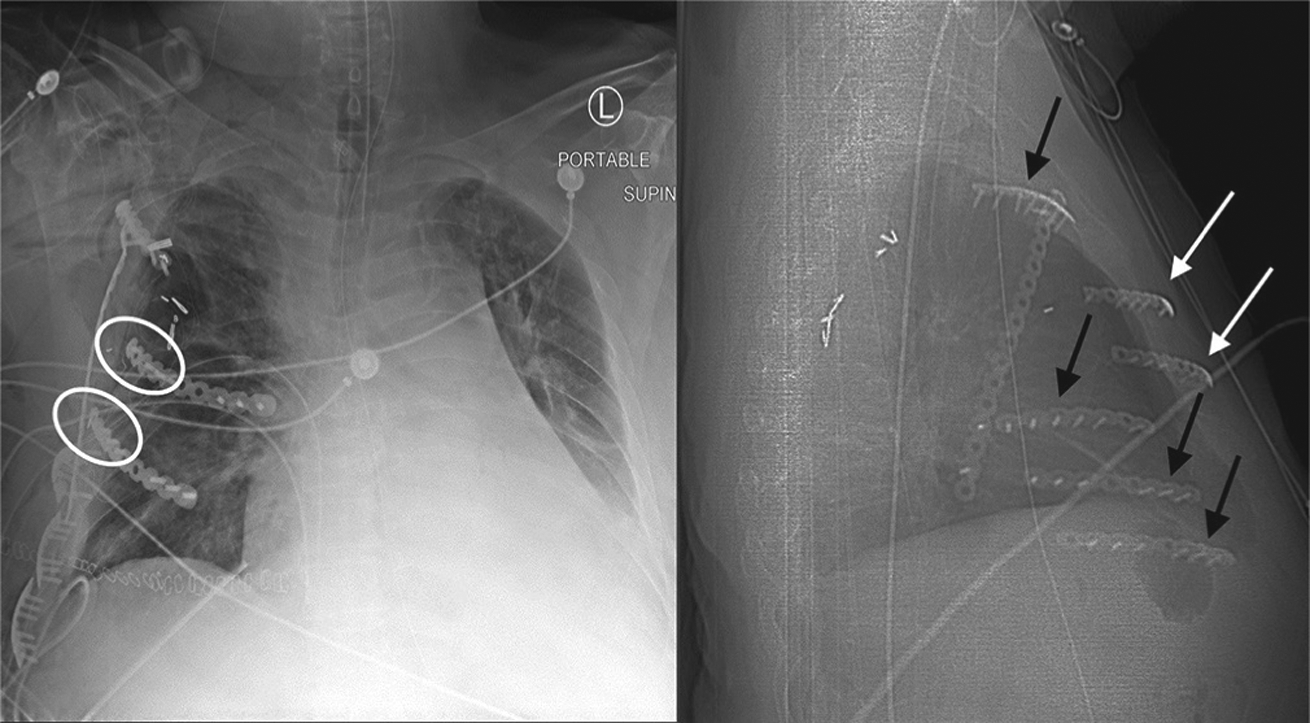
X-ray post repair. Horizontal plates spanning ribs 3 and 4 (white arrows), and ribs 2, 5, 6, and 7 (black arrows) are visualized. 1.0 Prolene was used to attach vertical plate to ribs 3 and 4 (circles)
